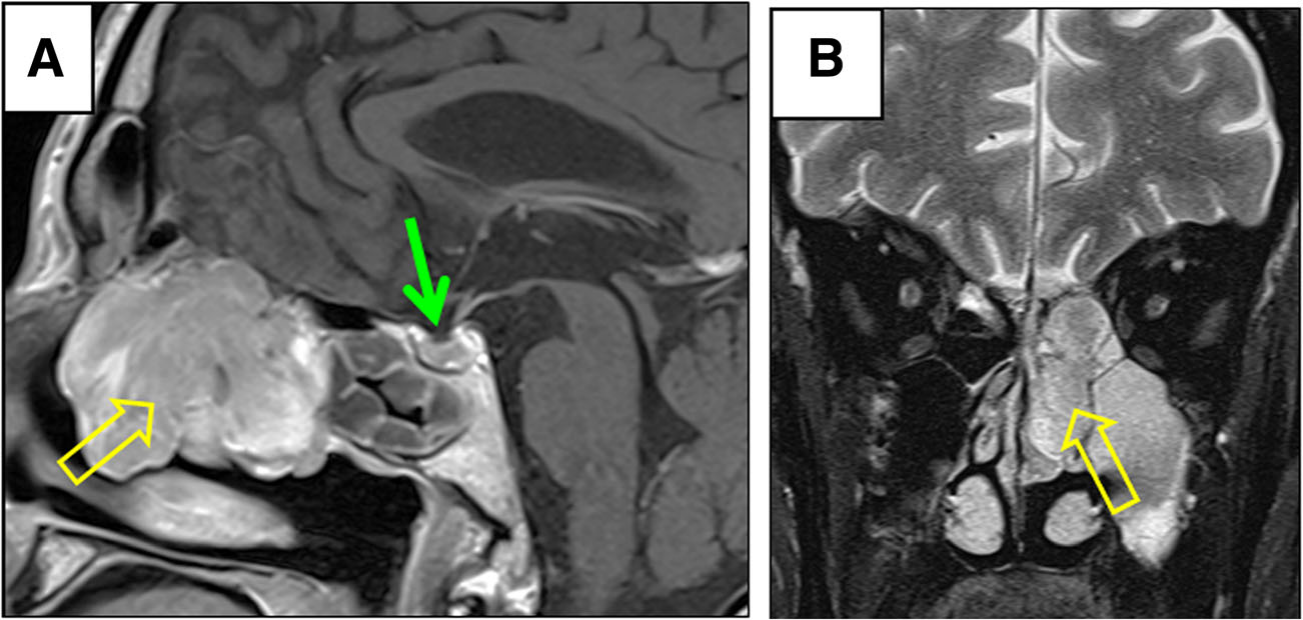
Sagittal T1-weighted MRI with gadolinium contrast (a) and Coronal T2-weighted MRI (b) revealing an enhancing nasal mass extending down from the cribriform plate of the anterior cranial fossa (open arrow), with the presence of a normal pituitary gland (solid arrow)
